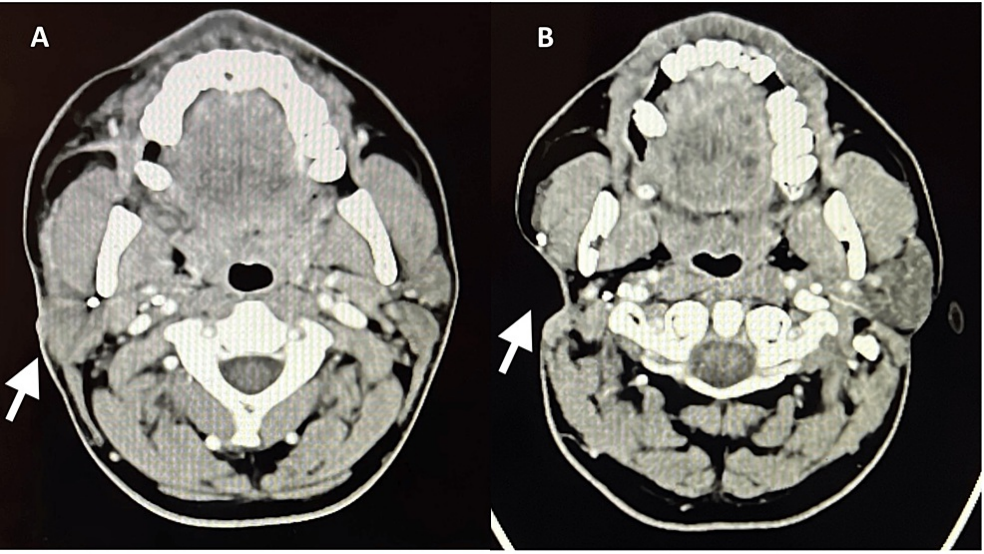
(A) CT scan, Arrow: shows right-sided parotid local recurrence. (B) CT scan, Arrow: area post revision parotidectomy.
